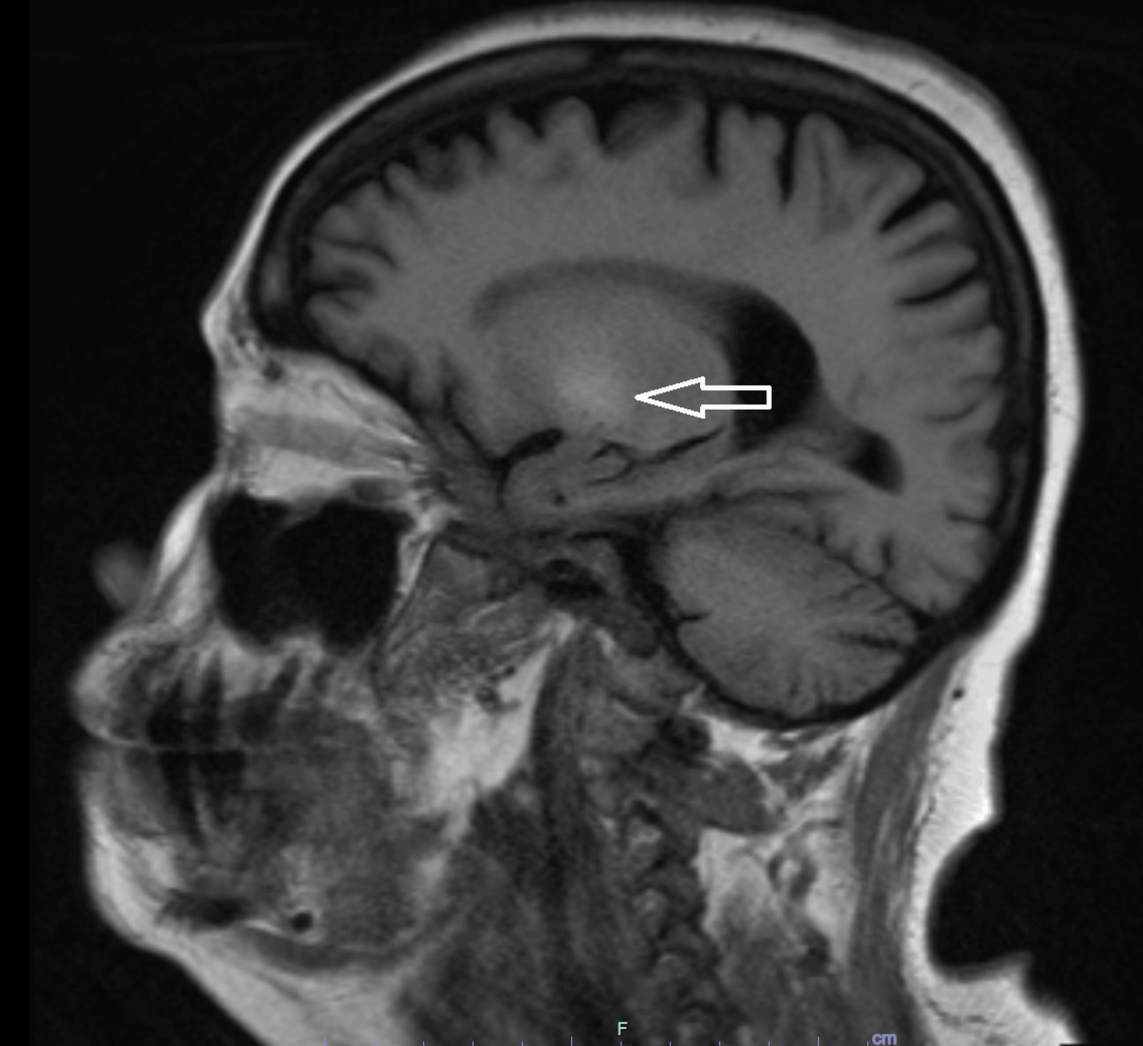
Sagittal T1-weighted MRI of the brain, with the arrow marking the globus pallidus hyperintensity
